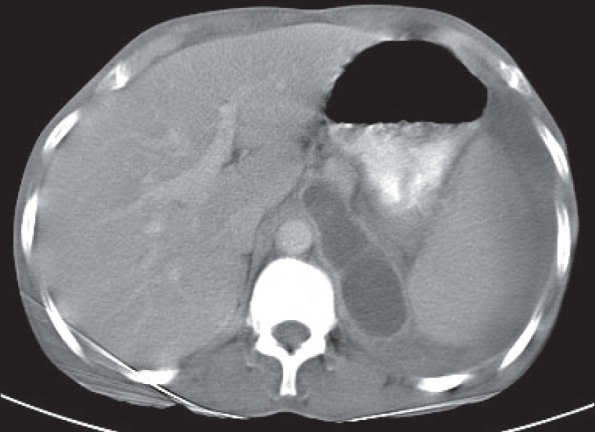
CT scan abdomen showing pancreatic pseudocyst
